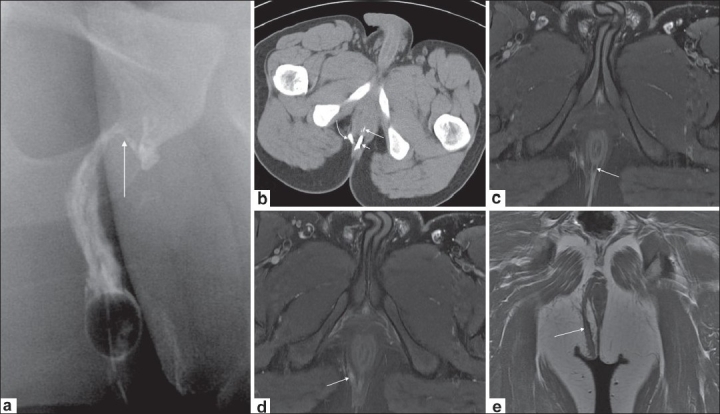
Combined trans- and extrasphincteric fistula. Conventional fistulogram (a) shows a right-sided large well-defined fistula (arrow). CT fistulogram (b) depicts contrast in the rectum (long arrow) with two closely located tracts, one lying close to the midline (short arrow) and the other being lateral (curved arrow) to the right external sphincter (extrasphincteric). The axial fat-saturated T2W images (c and d) reveal one tract to be transsphincteric (arrow in c) and the other to be extrasphincteric (arrow in d). Coronal T1W image (e) depicts the complete course of the extrasphincteric tract (arrow)
